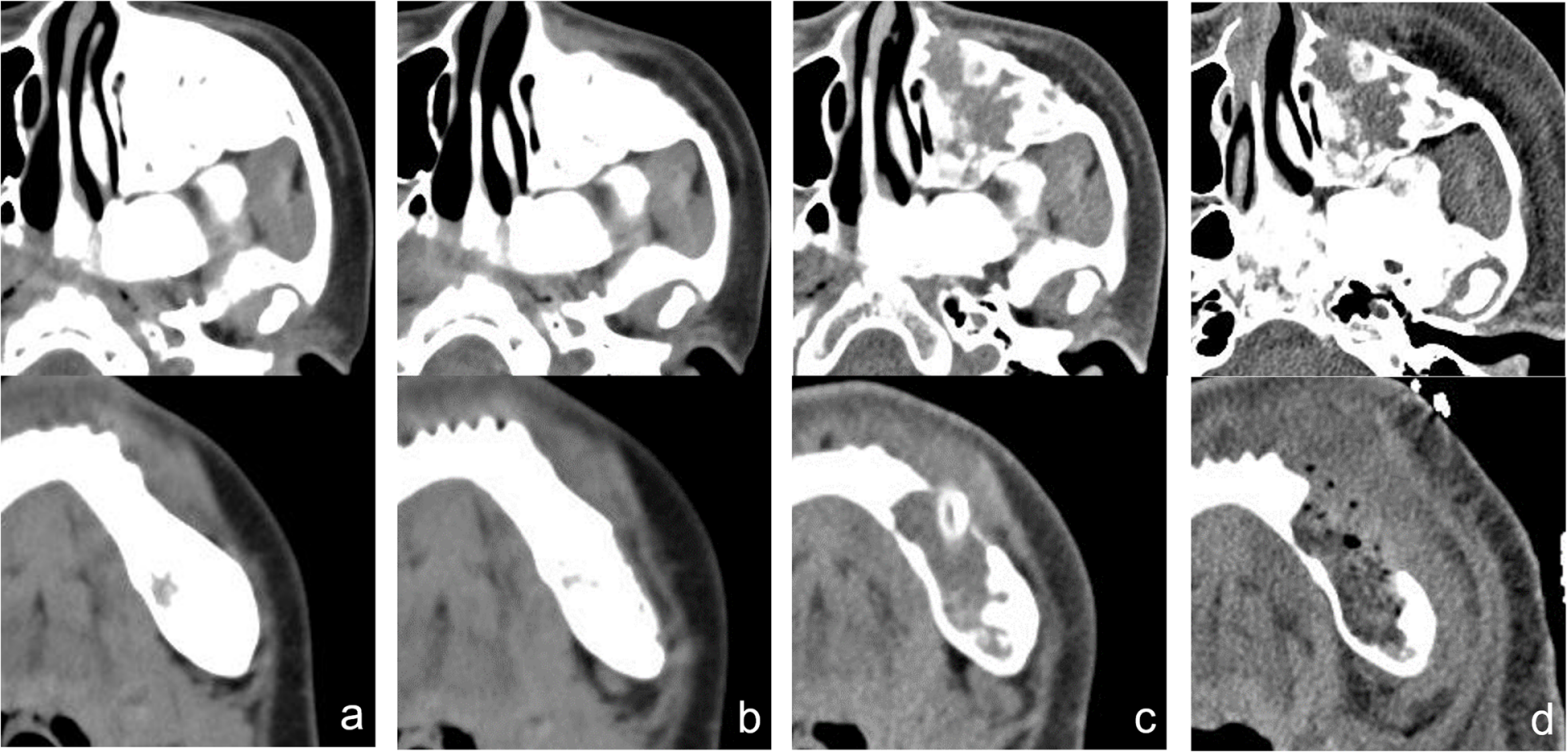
Contrast-enhanced facial computed tomography (CECT) images of the patient. a CECT image taken at the first visit to the out-patient department (2007.5). b CECT image taken 3 months postoperatively (2007.8). c CECT image after incisional biopsy and marsupialization (2018.11). d Postoperative CECT image after cyst enucleation on the left mandible in November 2018
